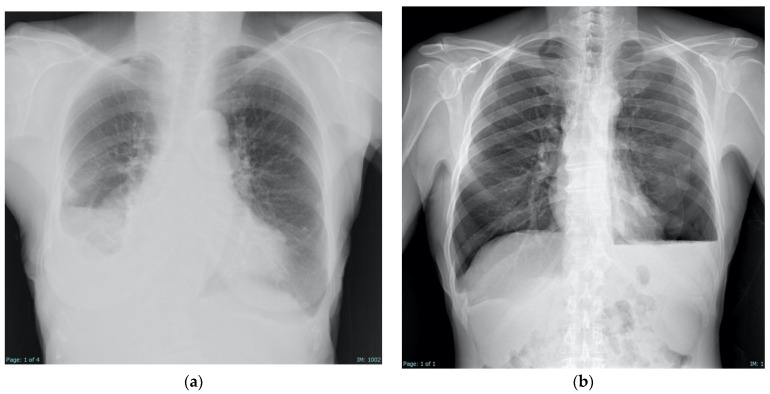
(a) Posteroanterior chest radiograph demonstrating hydropneumothorax in a patient with chronic multifactorial pleural effusion and non-expandable lung (NEL). (b) Posteroanterior chest radiograph showing hydropneumothorax following pleural drainage in a patient with malignant pleural mesothelioma.
